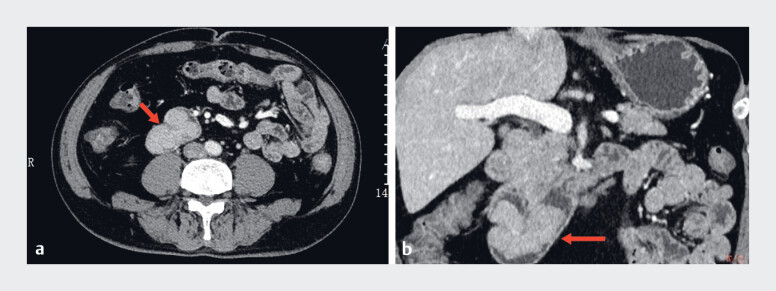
Preoperative computed tomography (CT) scan of the upper abdomen.
